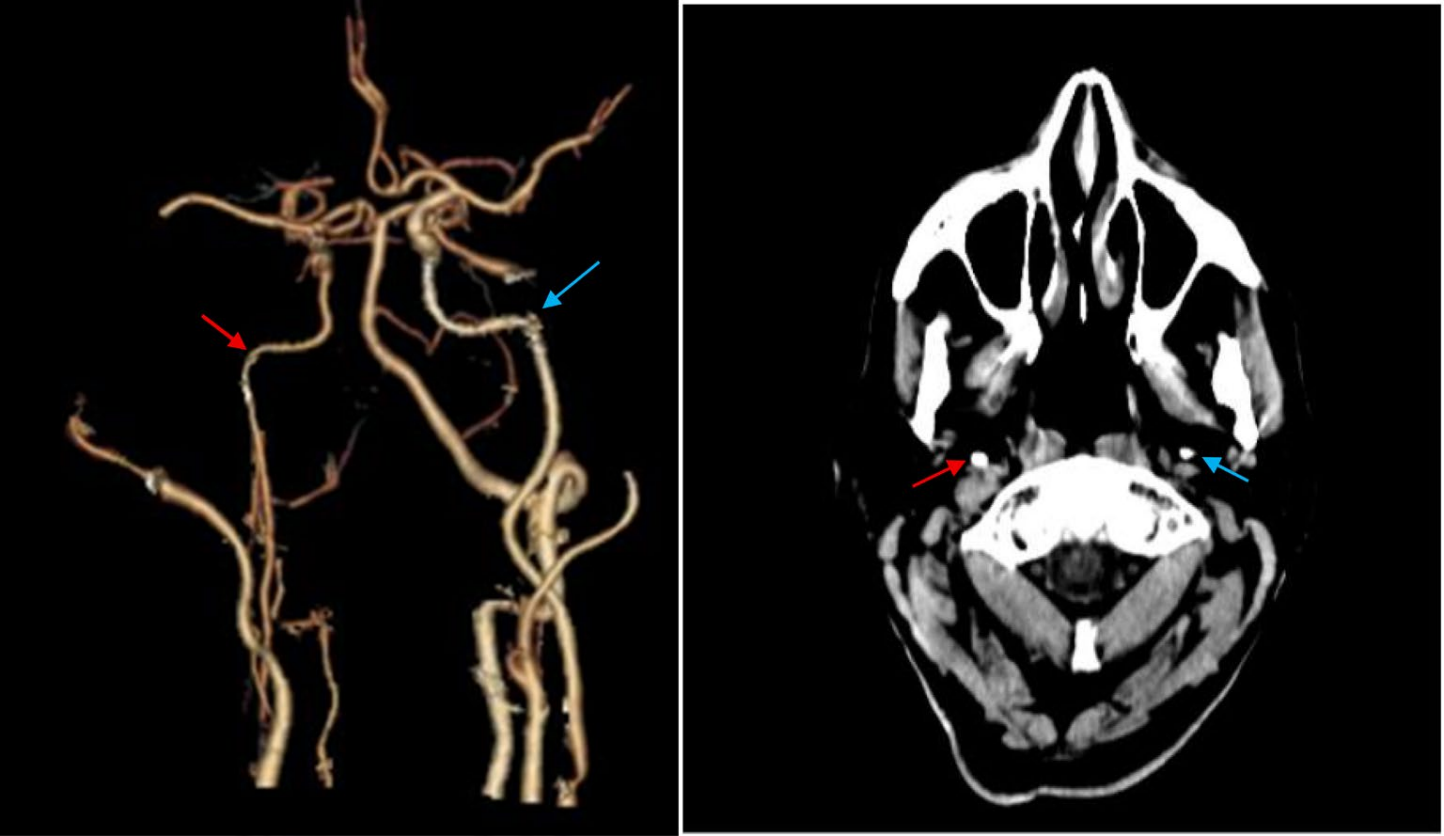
Postoperative CTA image of the right carotid artery demonstrating successful stent removal and complete vascular reconstruction (red arrow). No residual stenosis or complications are observed. Postoperative CTA image of the left carotid artery showing persistent 75% stenosis (blue arrow), which is scheduled for intervention in the second stage.
